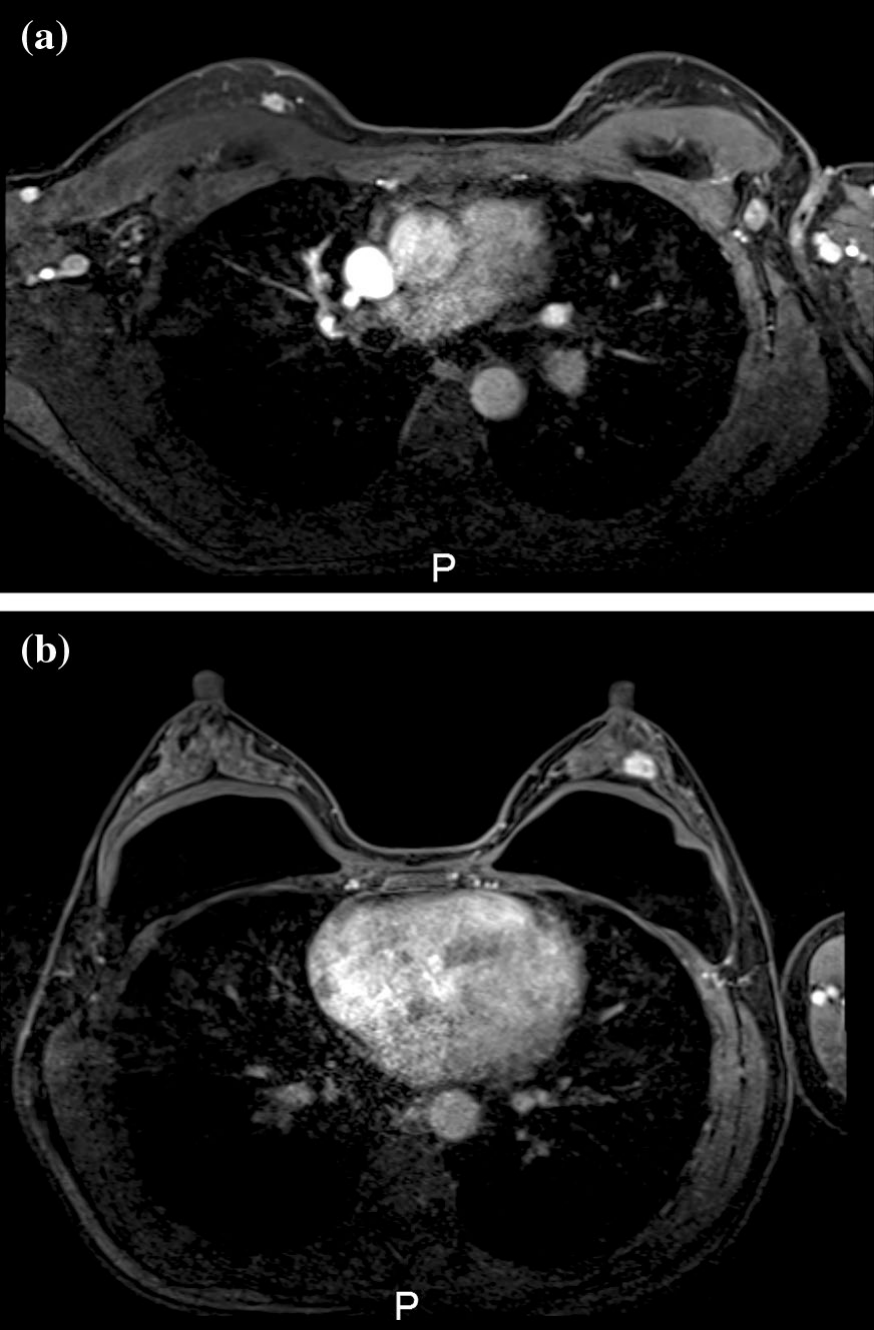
Magnetic resonance imaging (MRI). MRI showed enhancement of the lesion. There were implants under the pectoralis major muscle. a Right, b left
